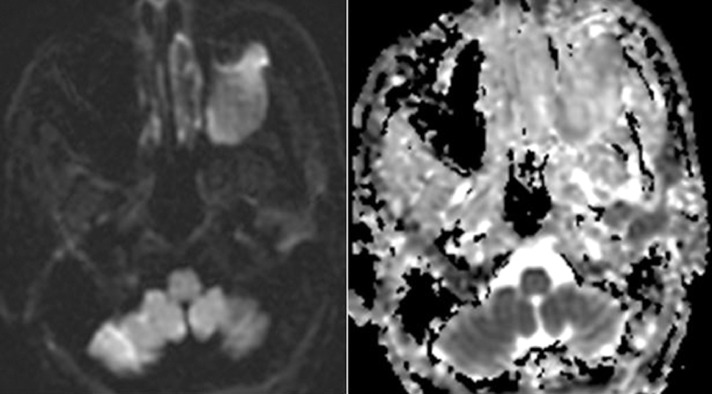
Axial diffusion-weighted images (DWI) and apparent diffusion coefficient (ADC) maps: No restricted diffusion is noted within the left maxillary soft tissue mass.
